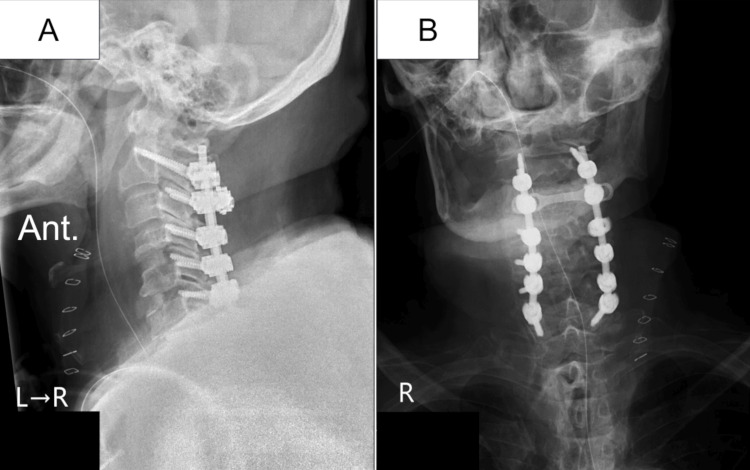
Radiographic images after the first operation. Anterior cervical discectomy and fusion of C4/5 and C5/6 and posterior cervical fixation from C2 to C7. The bone graft at C5/6 extruded anteriorly, however, postoperative dysphagia did not occur (A: lateral projection; B: anterior-posterior projection).
